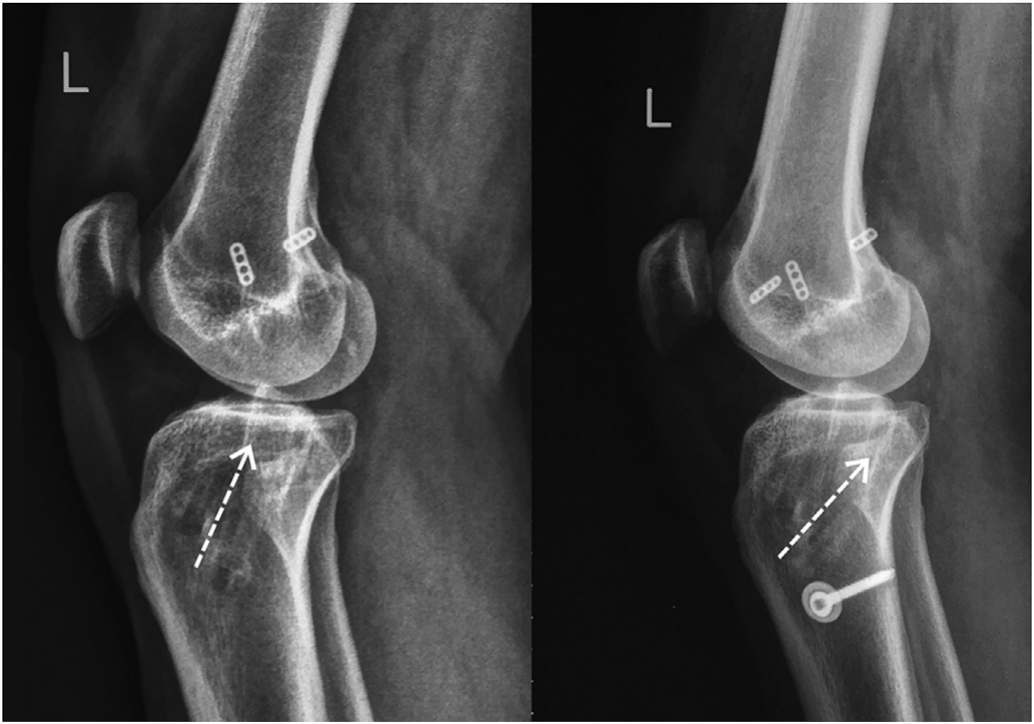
Comparative lateral x-ray before and after revision, tibial tunnel direction was replaced by new tunnel.
